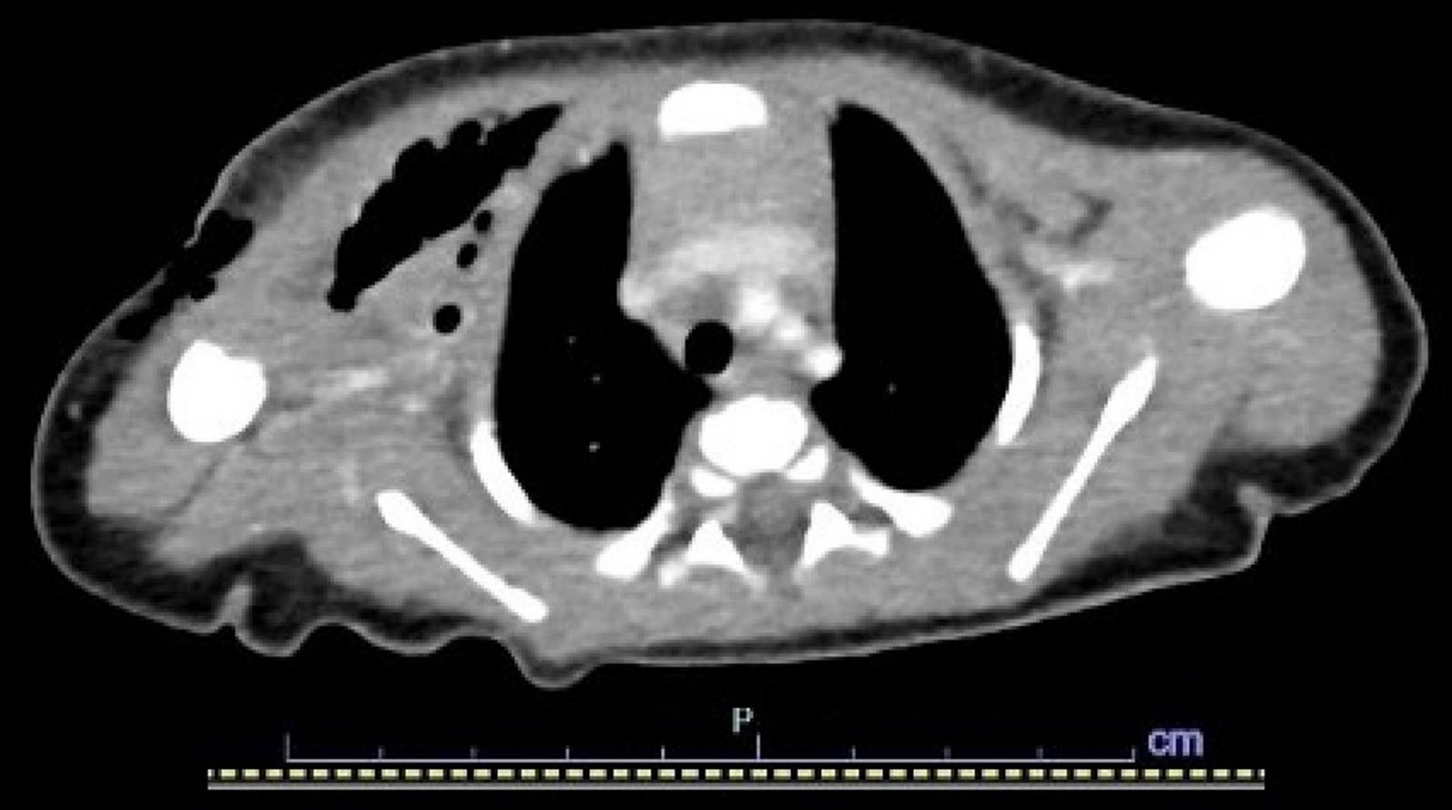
Preoperative contrast-enhanced CT immediately after removal of the PICC. CT shows emphysema in the subcutaneous area and between the muscles of the right anterior chest
